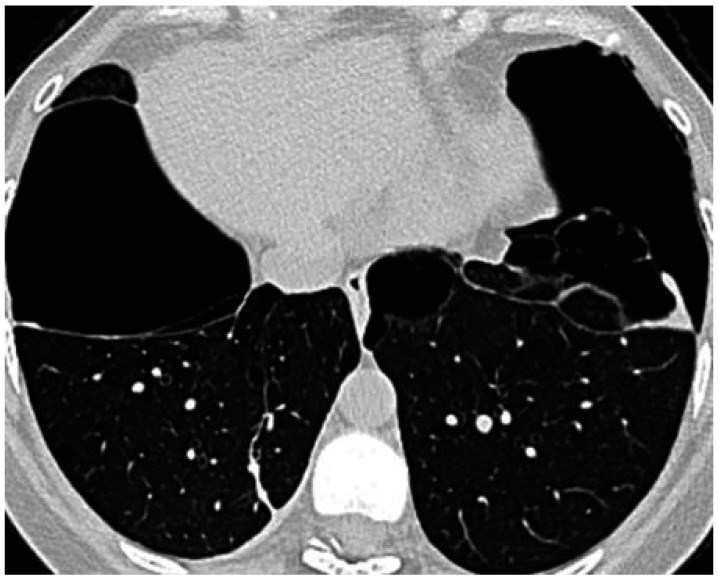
Chest CT, Case 5: left apico-parietal pneumothorax with bilateral cysts of varying shapes and sizes.
